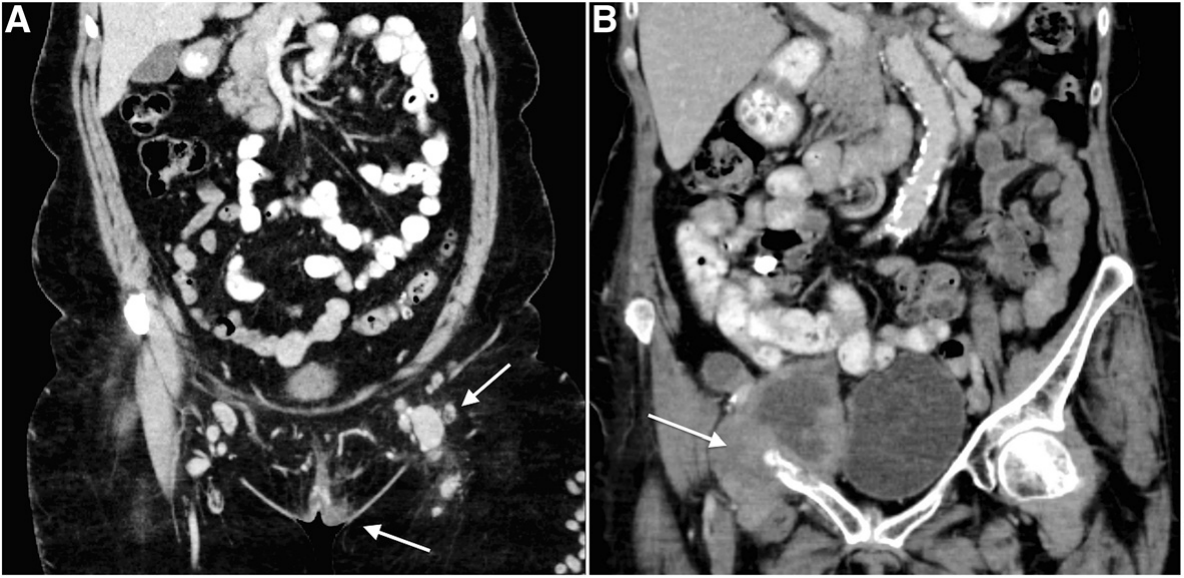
a A 56 year old woman with a left-sided vulvar carcinoma (*lower arrow*), palpable inguinal nodes and the corresponding CT scan showing metastases with enlargement and contrast enhancement (*upper arrow*). b A 74 year old woman with unexpected invasion of the ilium by lymph node metastases (*arrow*), clinically regarded resectable prior to surgery
